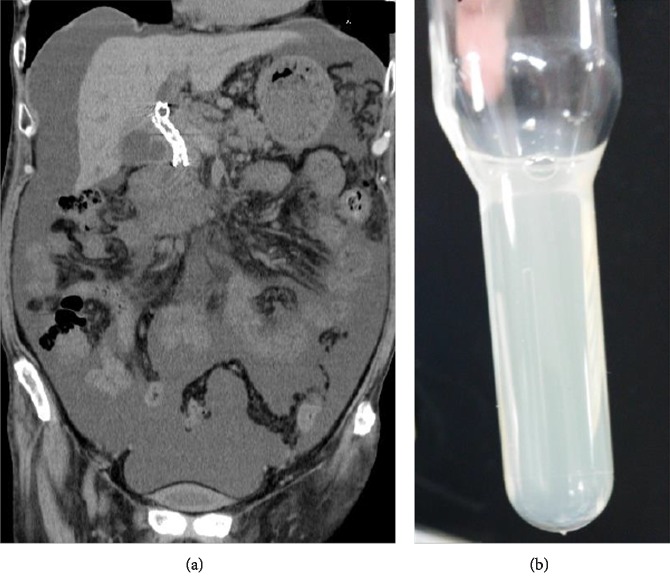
A large amount of ascites was stored in the abdominal CT (a). Harvested ascites (b).
